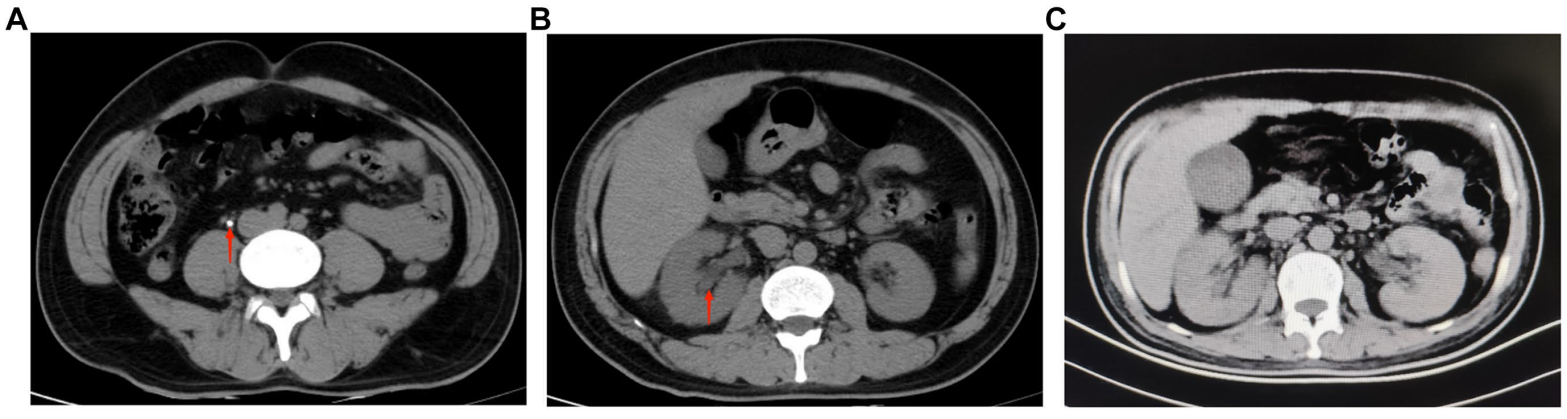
(A) Ureteroscopic lithotripsy preoperative abdominal CT showed a right upper ureteral stone (red arrow), about 0.5 cm in diameter (July 24, 2022). (B) Ureteroscopic lithotripsy preoperative abdominal CT showed no significant abnormality in the morphology, size and density of both kidneys, and clear perinephric space in both kidneys. The red arrow shows mild dilatation of the right renal pelvis (July 24, 2022). (C) The patient was transferred to our department for the first abdominal CT. The images showed no significant abnormalities in both kidneys and bilateral ureters (September 15, 2022, day 12 of admission).
